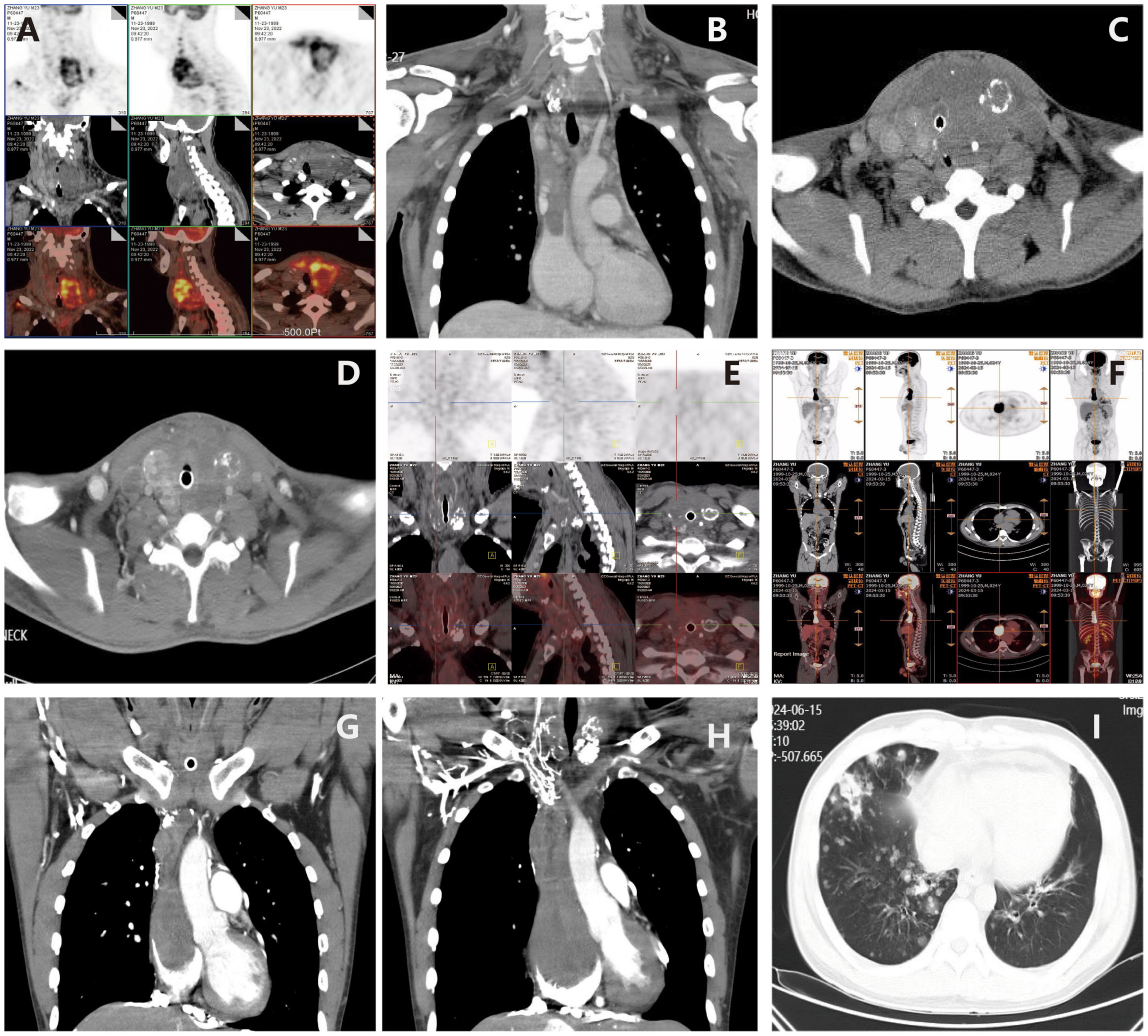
Imaging examination of thyroblastoma. (A, B) (November 23, 2022) PET/CT revealed irregular high-metabolic masses in the thyroid gland (more prominent in the left lobe) with calcification, compressing the trachea, causing local tracheal narrowing and rightward deviation; Multiple thromboses and cancerous thrombi were found in the superior vena cava, bilateral brachiocephalic veins, bilateral internal and external jugular veins, and the right subclavian vein. (C) (November 30, 2022) CT suggested rapid progression of thyroid tumor; (D) After two cycles of BEP regimen chemotherapy, enhanced CT (December 27, 2022) showed that the thyroid tumor had shrunk, the trachea was centered, and subcutaneous edema had decreased. (E) On August 25, 2023, follow-up PET/CT showed multiple low-density nodules with calcification in the thyroid, which were significantly smaller in volume than before, and metabolism had basically returned to normal, indicating that tumor activity was largely suppressed after treatment. The previously high-metabolic lesions in the superior vena cava and left brachiocephalic vein had returned to normal. (F) On March 15, 2024, PET/CT showed that the size of the thyroid lesion was unchanged and metabolism remained normal, suggesting suppressed tumor activity after treatment; however, new high-metabolic lesions in the superior vena cava and right atrium suggested cancer thrombi. (G) On March 19, 2024, CTA showed cancer thrombus formation in the lower lobe pulmonary arteries of both lungs, the right atrium, and the superior vena cava and its branches. (H) On June 12, 2024, CTA showed that cancerous thrombi had increased in size in the right atrium, right ventricle, superior vena cava, and left brachiocephalic vein. (I) On June 15, 2024, CT revealed multiple metastatic tumors in both lungs.
